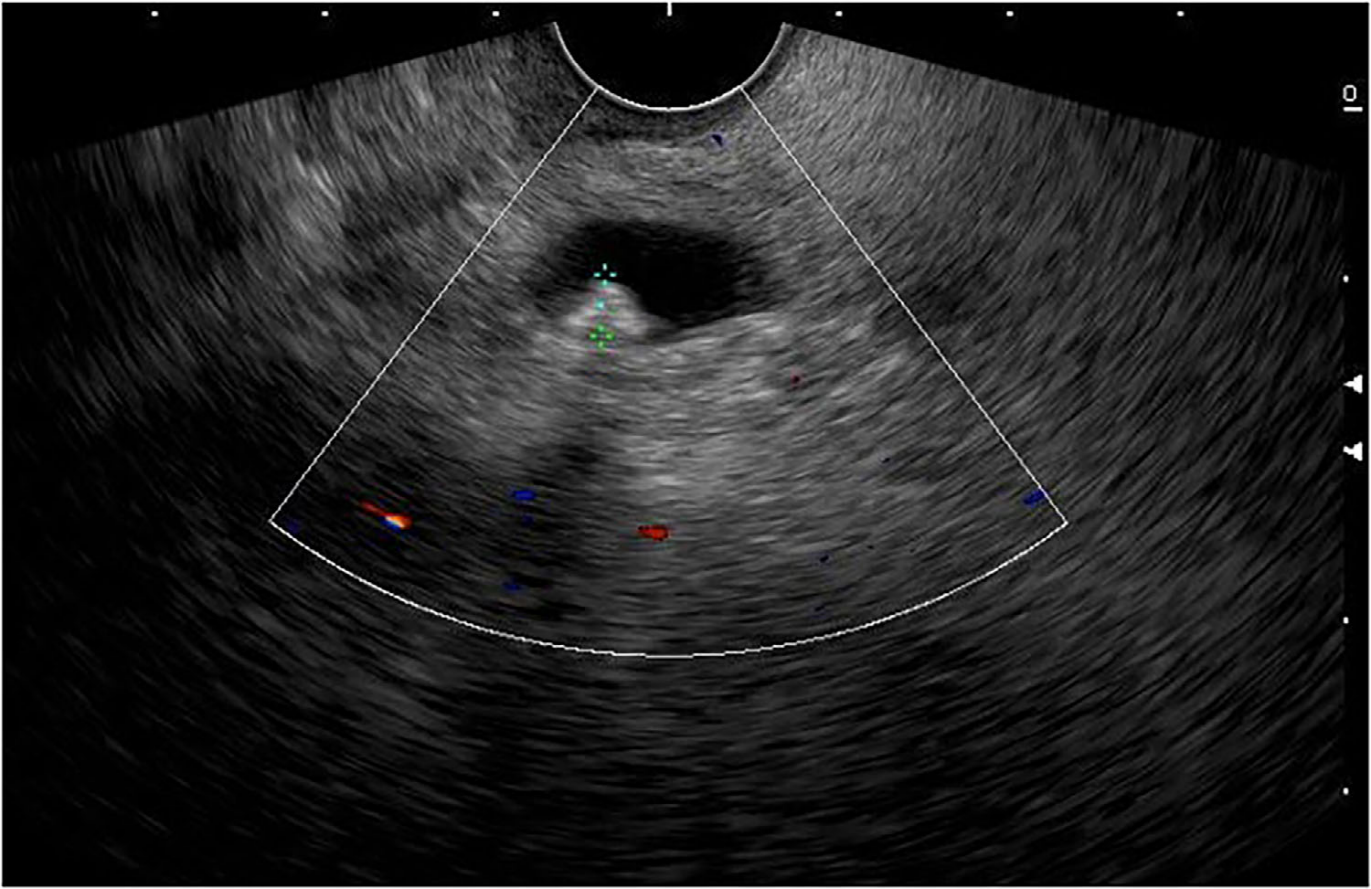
An endoscopic ultrasound (EUS) image revealing a single stone in a dilated common bile duct.
